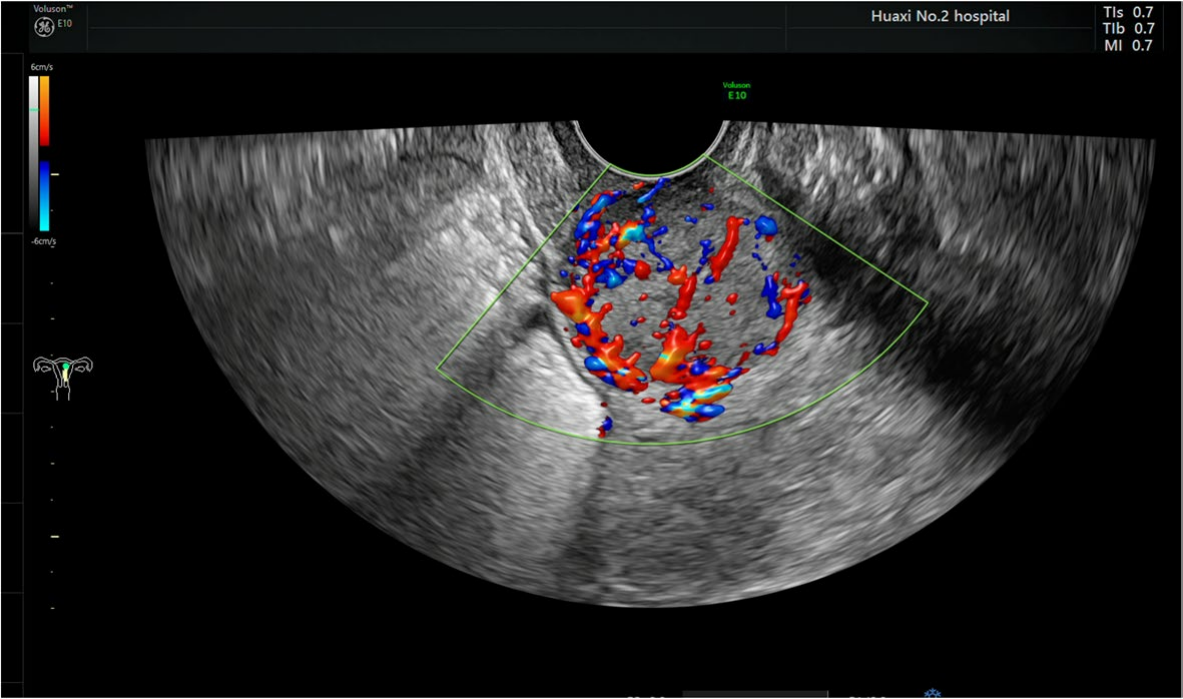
Transvaginal ultrasound examination showed an echogenic mass measuring 3 cm × 2.6 cm × 2.7 cm in the cervix with increased vascularity
